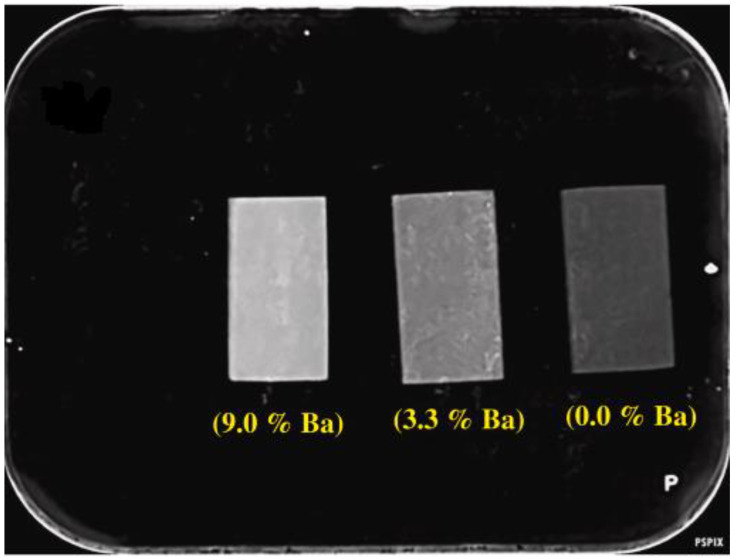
Radiograph of SiO_2_–CaO–P_2_O_5_–B_2_O_3_–Na_2_O–Al_2_O_3_ glass samples with 0% BaO, 3.3% BaO and 9% BaO (in mol%) [154].
